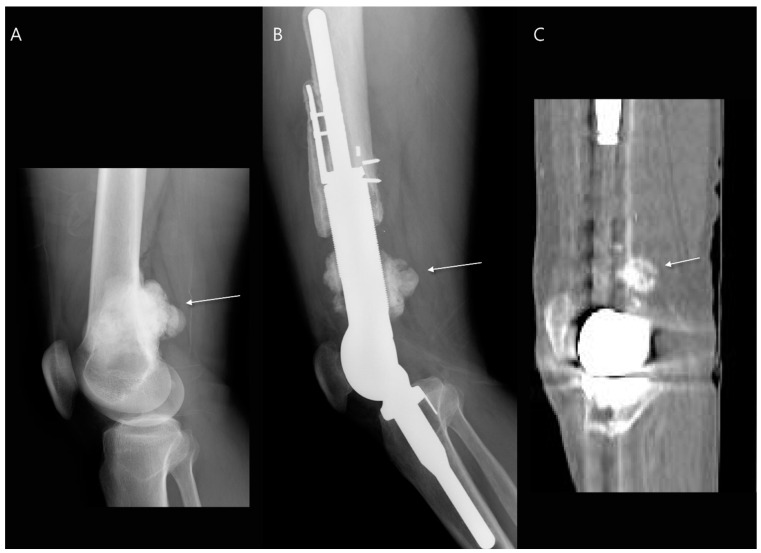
Local recurrence after limb-salvage surgery for parosteal osteosarcoma. (A) Preoperative radiograph reveals juxtacortical mineralization arising from the posterior aspect of the distal femur (arrow), consistent with a diagnosis of parosteal osteosarcoma. (B,C) One year later, both the radiograph and CT scan demonstrate mineralization surrounding the femoral component (arrows), indicative of recurrent osteosarcoma.
